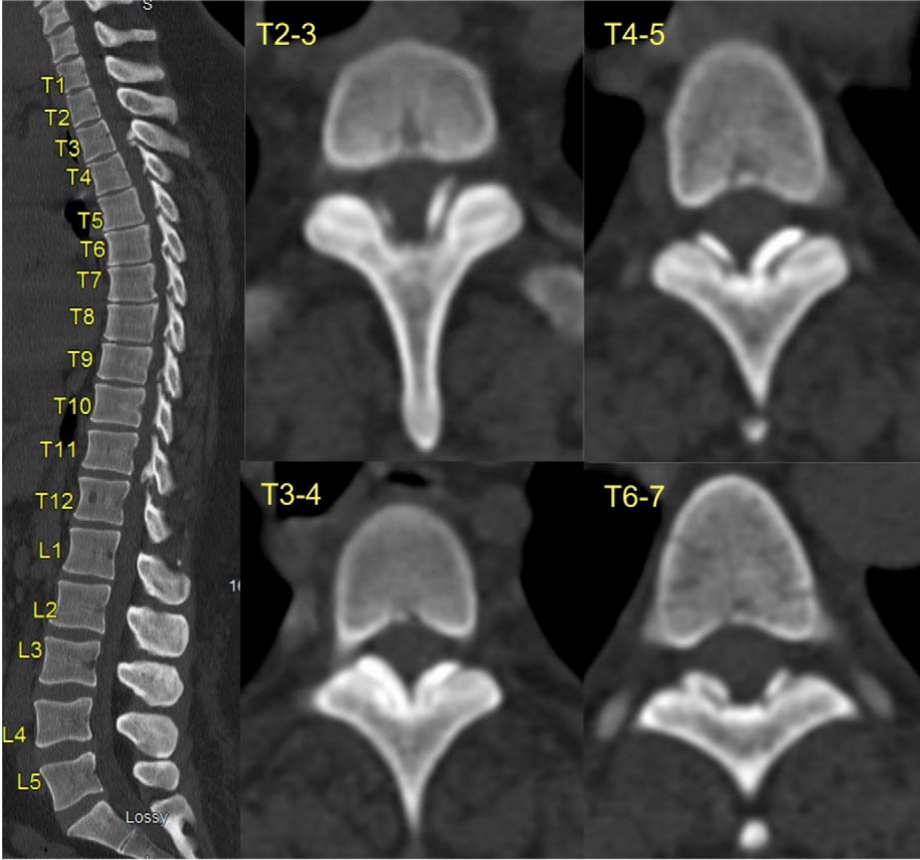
Sagittal (left) and axial (right) computed tomography cuts (T2-T7) of the thoracic spine showing segmental calcific lesions causing severe spinal stenosis.
